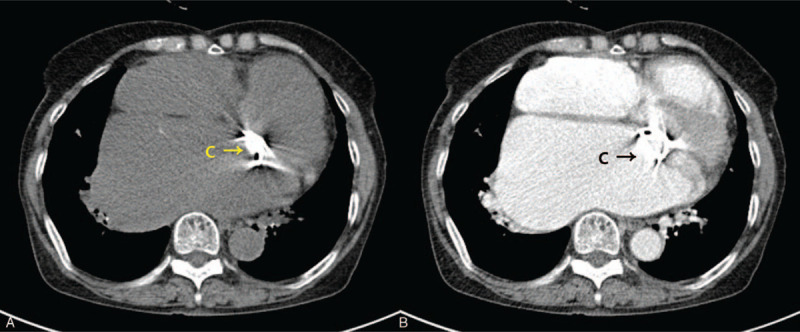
Preoperative CT scans revealed an enlarged atrium and a mechanical valve, but no specific findings in the left atrium. (A) Pre-enhanced CT scan at the pulmonary vein level. (B) Enhanced CT scan at the pulmonary vein level. (C) Previously inserted mitral prosthetic valve. CT = computed tomography.
